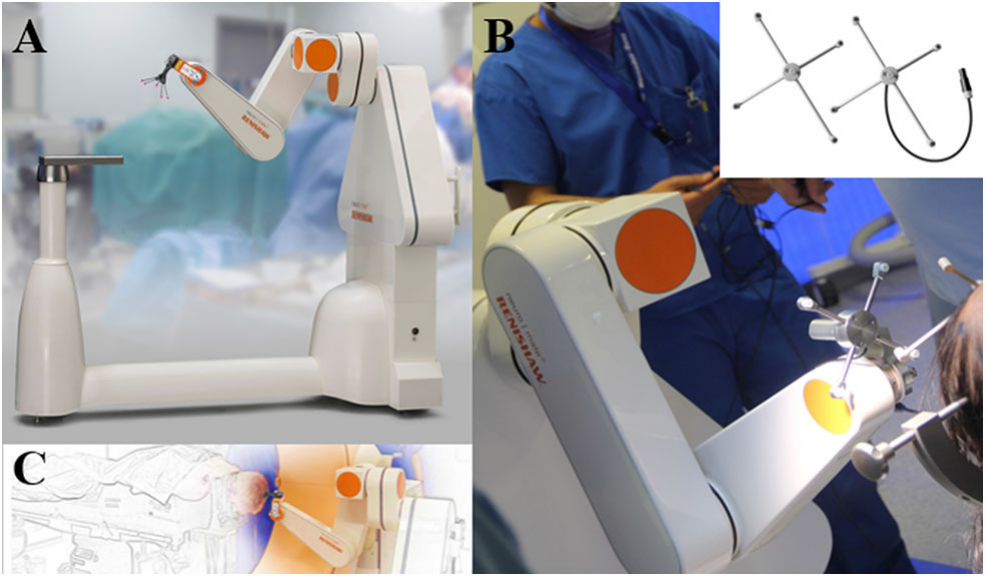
Neuromate-Robot system by Renishaw. (A,C) Neurolocate® system used for frameless registration. (B) Registration with Ultrasound technology. Images provided by RENISHAW with permission for publication (Renishaw-mayfield; Nyon, Switzerland).
